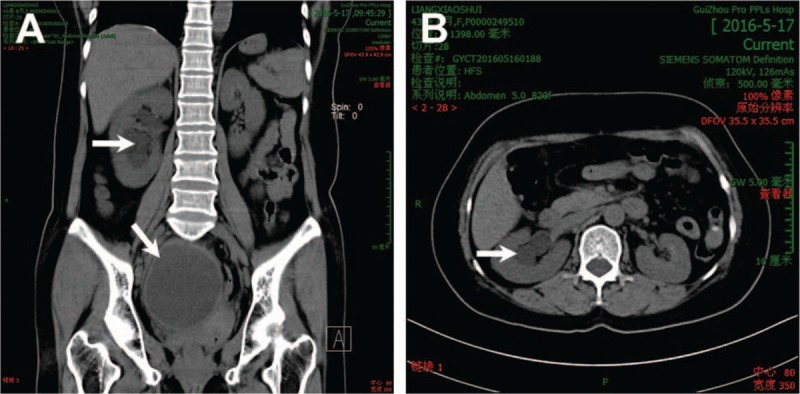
Computed tomography (CT) findings of idiopathic retroperitoneal fibrosis. CT showing soft-tissue low-density mass surrounding the right adnexa and right-sided hydronephrosis. CT = computed tomography.
